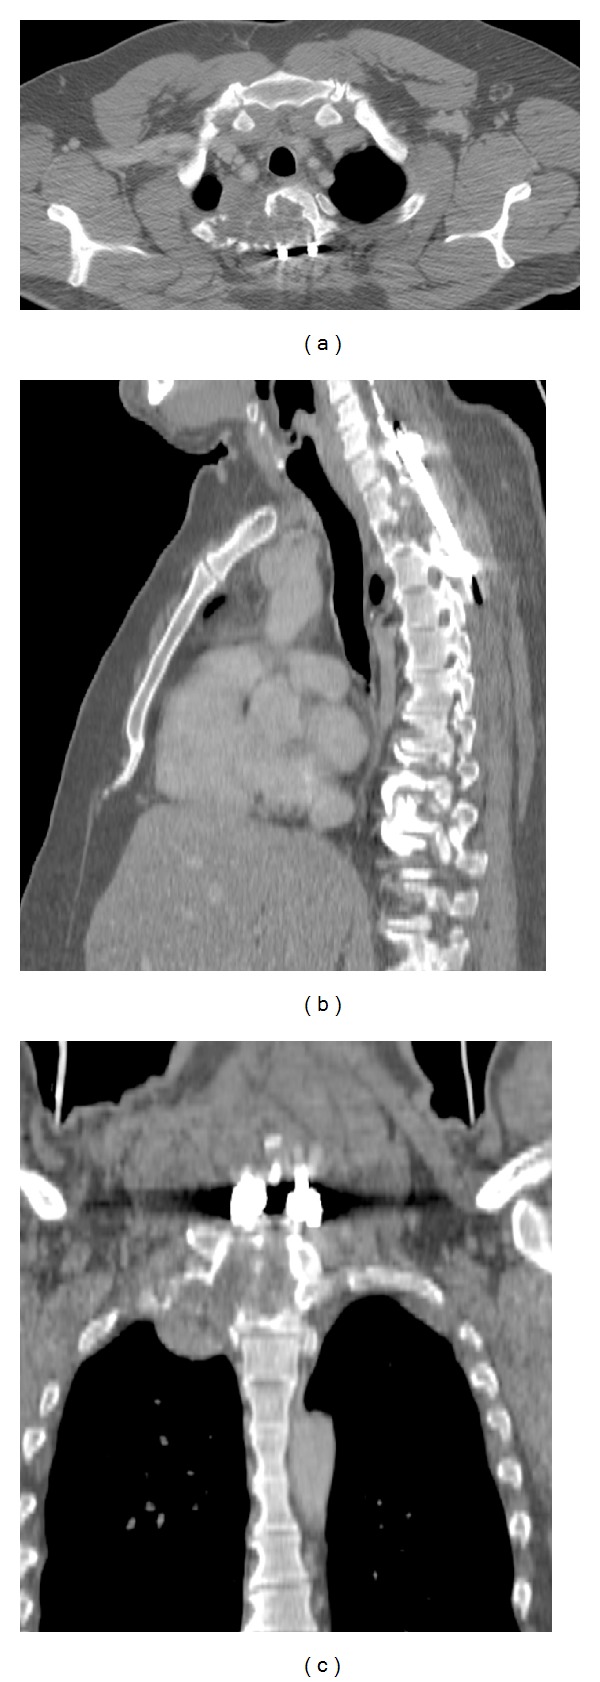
CT exam axial, sagittal, and coronal planes (a), (b), and (c) reveal a recurrence at the level of D1 and D2 vertebrae and the presence of cystic tissue in the chest on the right.
